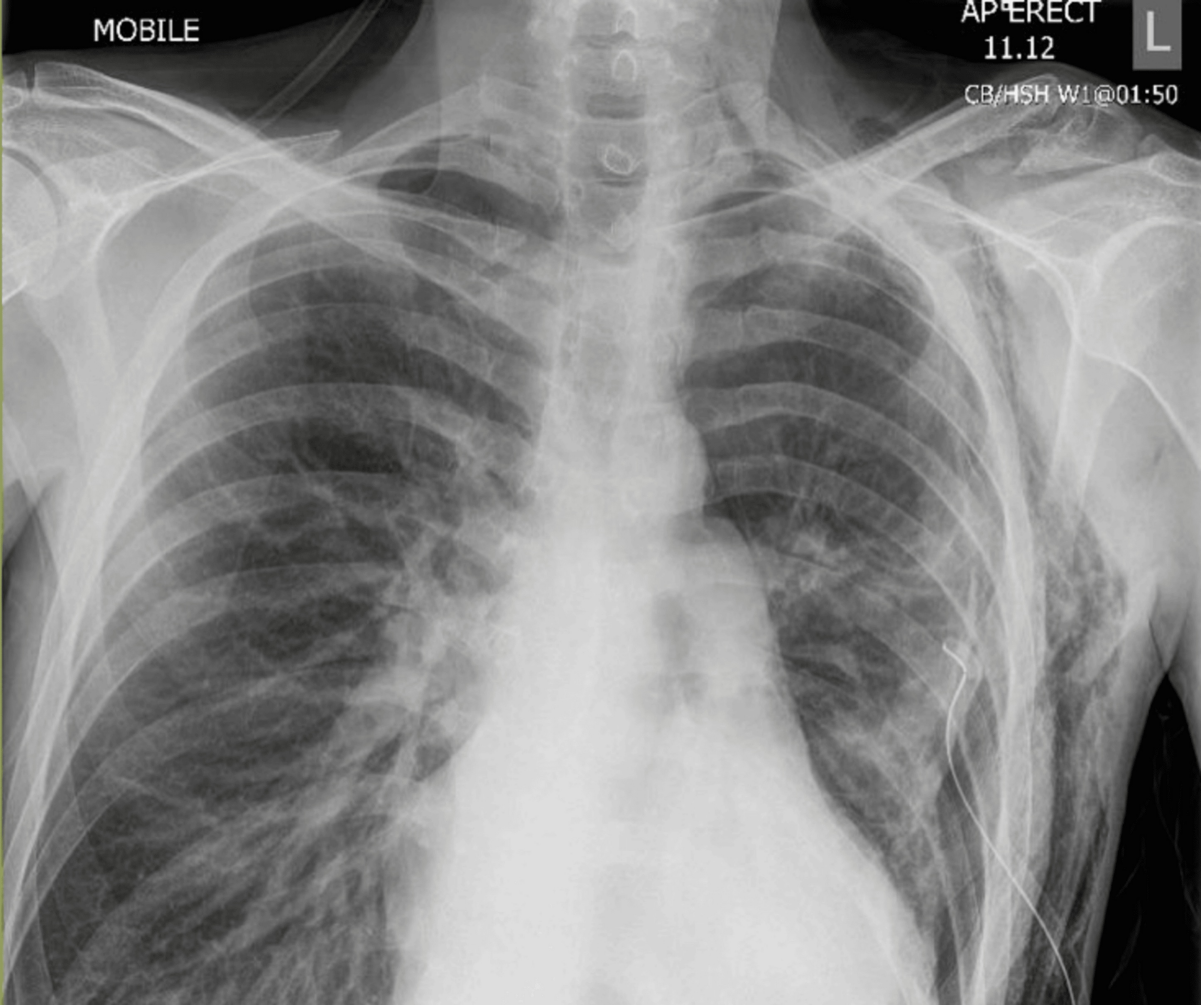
Chest X-ray showed the refractory left-sided pneumothorax despite chest drain insertion day 5
